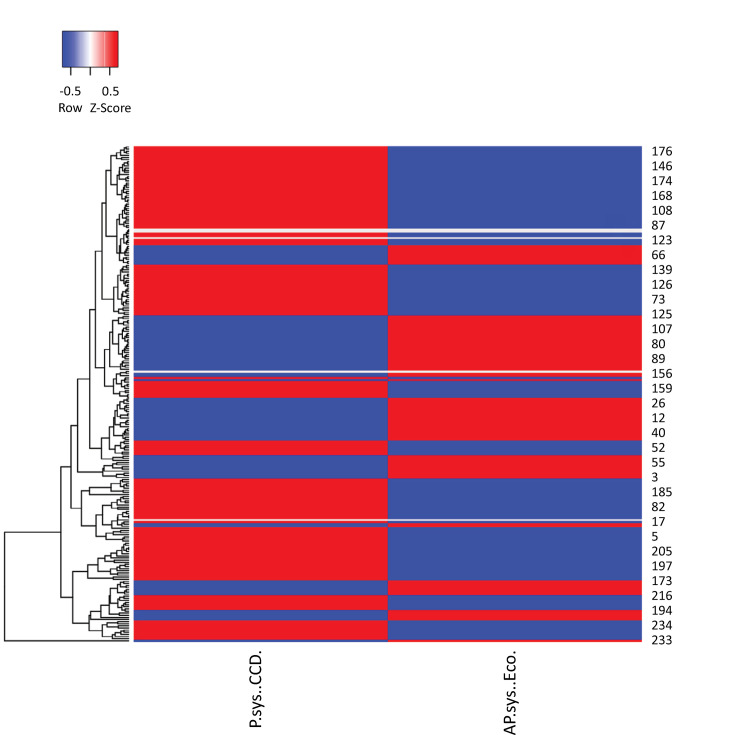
Heat plot for pulmonary artery systolic pressure estimated by transthoracic echocardiography and measured by right heart catheterization. The left sidebar corresponds to the dendrogram generated via an average clustering method with a Euclidean distance measure. PASP - pulmonary artery systolic pressure; RHC - right heart catheterization; TTE - transthoracic echocardiography.
